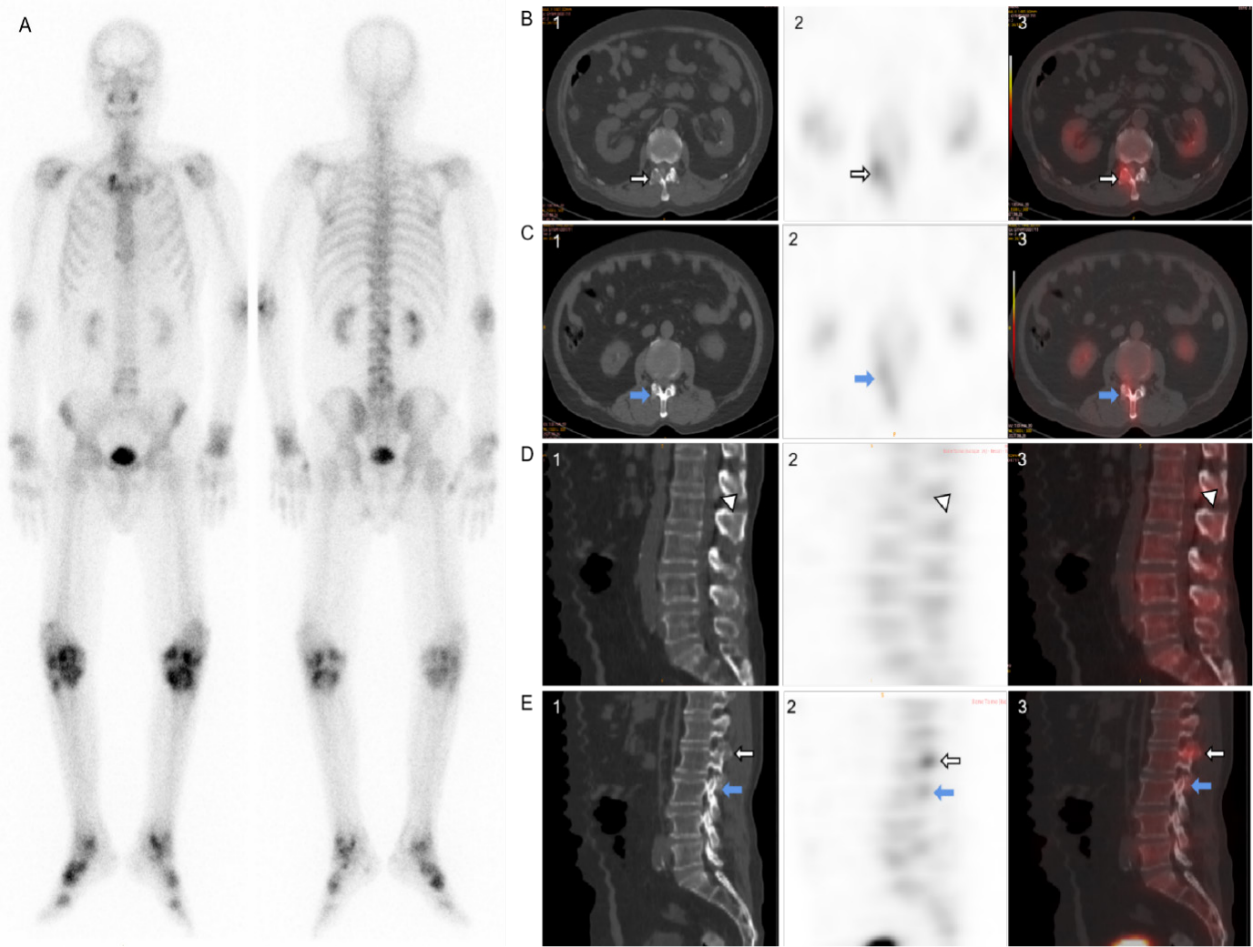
A 66-year-old elderly male. Postoperative pathological diagnosis of the lumbar spine: gout nodules. Anterior and posterior whole body views of ^99m^Tc-MDP skeletal scintigraphy are shown. (A) Multiple regions of anomalous uptake of ^99m^Tc-MDP were detected in the lumbar 1/2 right, right rotoclavicular, knee and ankle joints. (B–E) SPECT/CT can synchronize the case of bone, which enables the acquisition of synchronous imaging information of metabolism (focal anomalous uptake of ^99m^Tc-MDP can be seen in an intra-spinal canal nodule at level L1/2 [white triangle]) and bone destruction in the gouty lesion area (bone erosions can be seen in the right inter-apophyseal joints at levels L1/2 [white arrow] and L2/3 [blue arrow]).^[64]^ MDP: methylene diphosphonate; SPECT: single photon emission computed tomography; CT: computed tomography.
